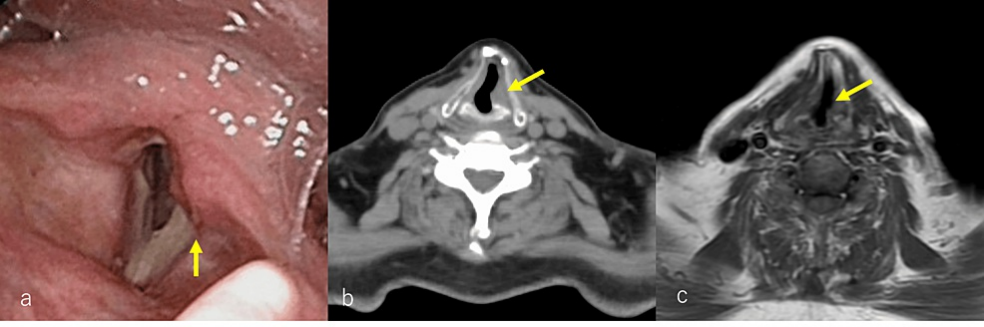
Laryngoscopy, CT, and MRI of the patient. (a) On laryngoscopy, it was observed that the left vocal folds were fixed during inspiration (yellow arrow). (b) CT scan revealed a left-right difference near the vocal folds (yellow arrow). (c) In MRI T1-weighted imaging, a left-right difference near the vocal folds was also indicated (yellow arrow).
